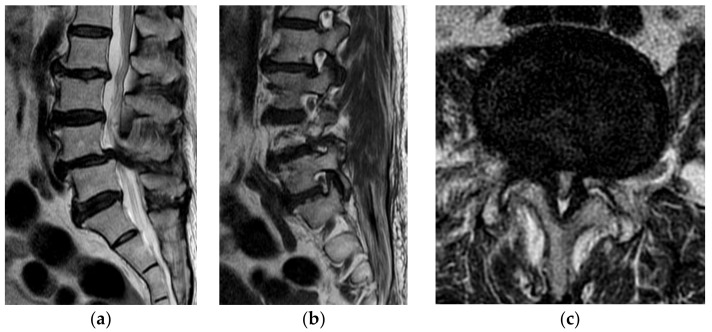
A 71-year-old female presented with severe radiating pain in both legs, with claudication. (a) The sagittal MR image shows central canal stenosis with spondylolisthesis at the L4–5 level. (b) The right parasagittal MR image shows severe foraminal stenosis. (c) An axial MR image shows severe central canal stenosis at the L4–5 level. Facet hypertrophy, thickening of the ligamentum flavum, and the bulging disc severely compress the dural sac.
